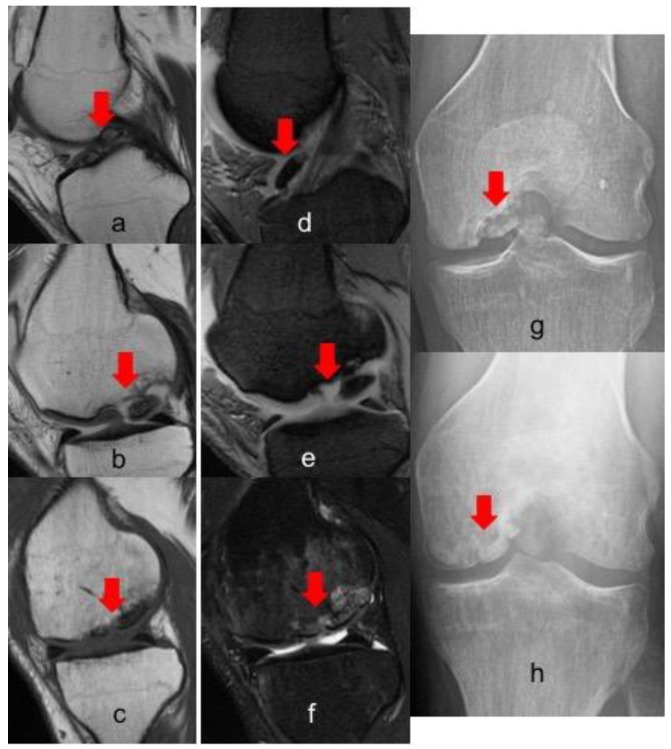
MRI and X-ray evolution of a grade IV OCD of 4.6 cm^2^ in the medial condyle of a right knee fixed with three Smart Nails. (a,b) T1 preoperative sagittal view; (d,e) T2 preoperative sagittal view; (c–f) 1 year postoperative sagittal view; (g) preoperative X-Ray; (h) 5 years postoperative X-ray. In all images the red arrow indicates the lesion, in figure a-d the red flag indicates a free intra-articular body.
